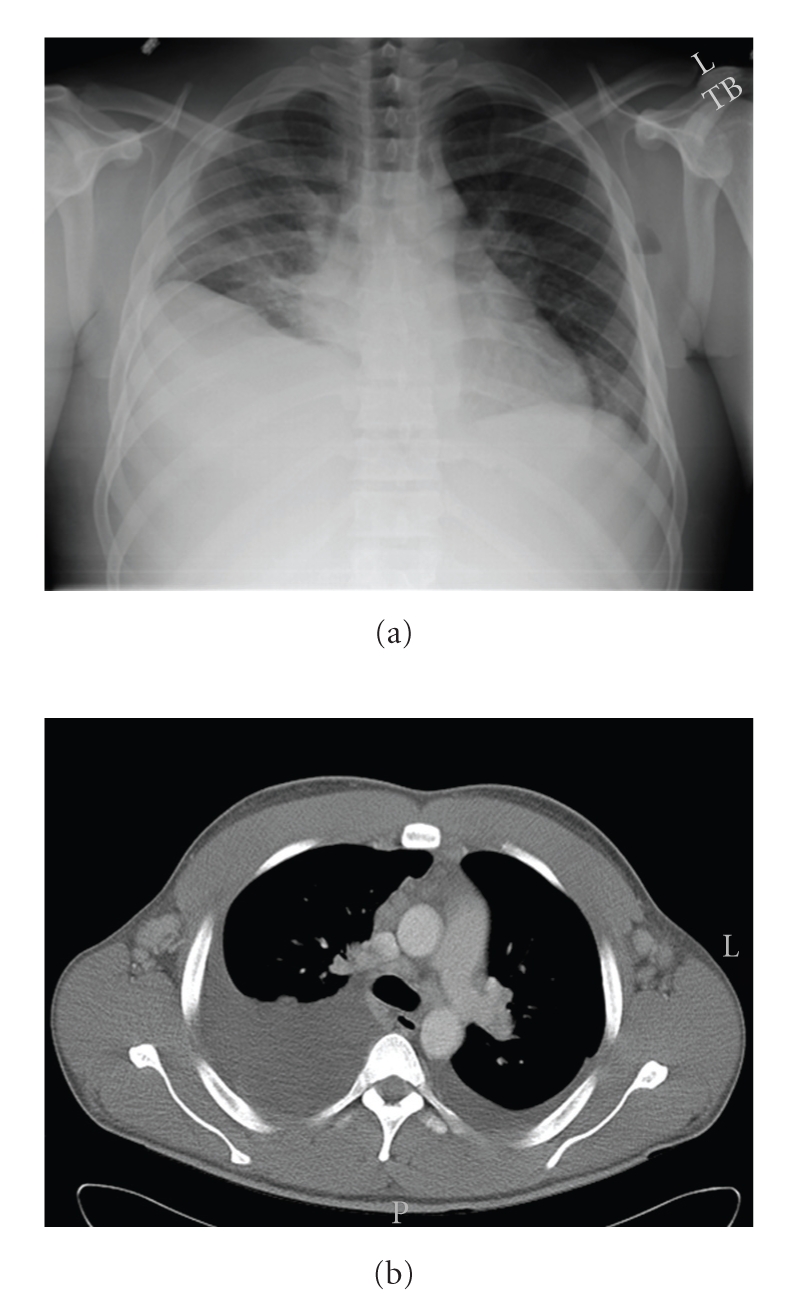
Chest radiography and CT scan of the chest showing bilateral pleural effusions.
